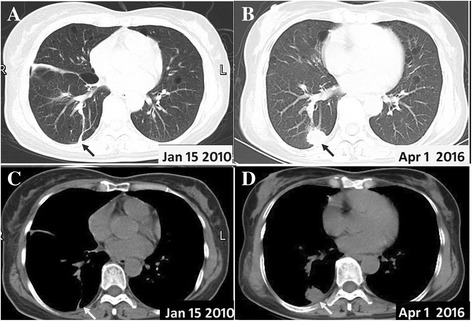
Chest CT scan with pulmonary pseudotumor. a Previous CT scan shows a fibrous change in the same slice. b The present CT scan shows a 2.8 cm × 2.3 cm pulmonary solid lesion with irregular margins and adjacent pleural thickness. c, d Corresponding mediastinal window
